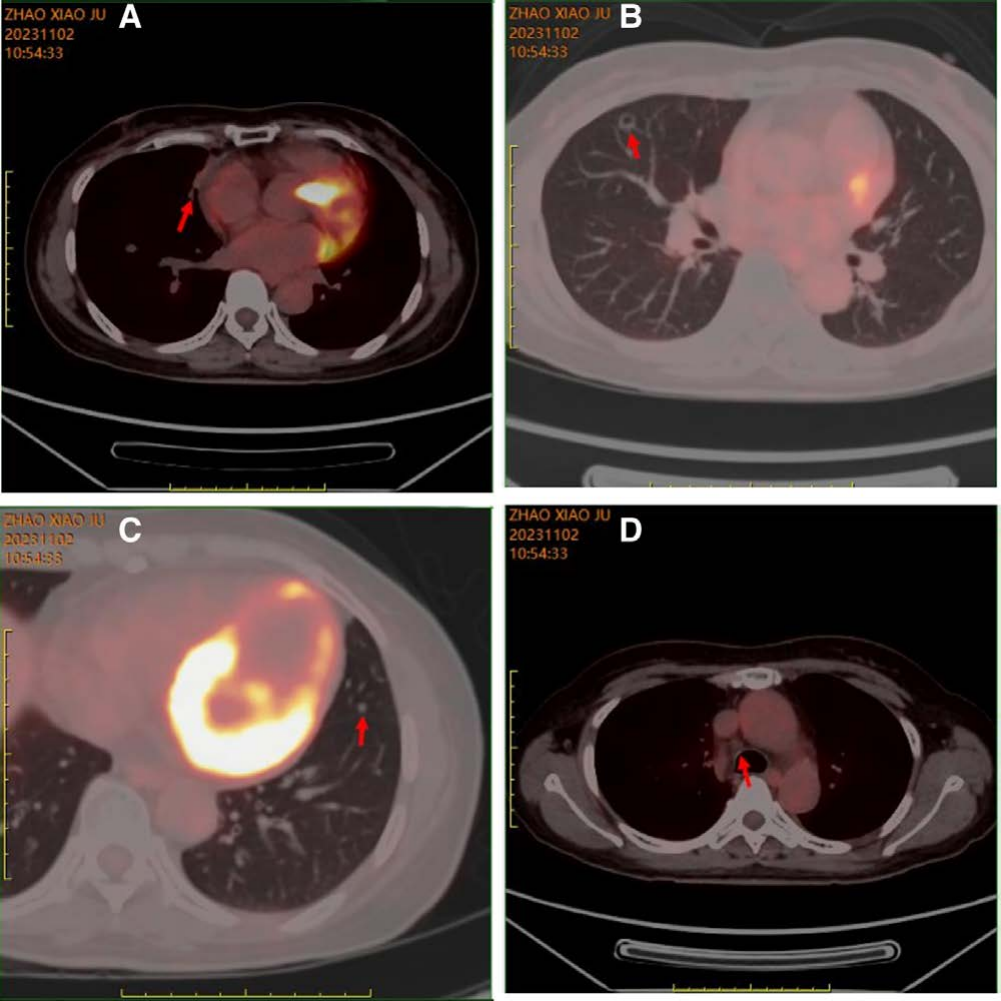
PET-CT Results on November 2, 2023. (A) No increase in the size of the primary lesion; (B) Stable treated metastatic lesion; (C) Stable treated metastatic lesion; (D) No enlargement of the mediastinal lymph nodes. CT = computed tomography, PET = positron emission tomography.
